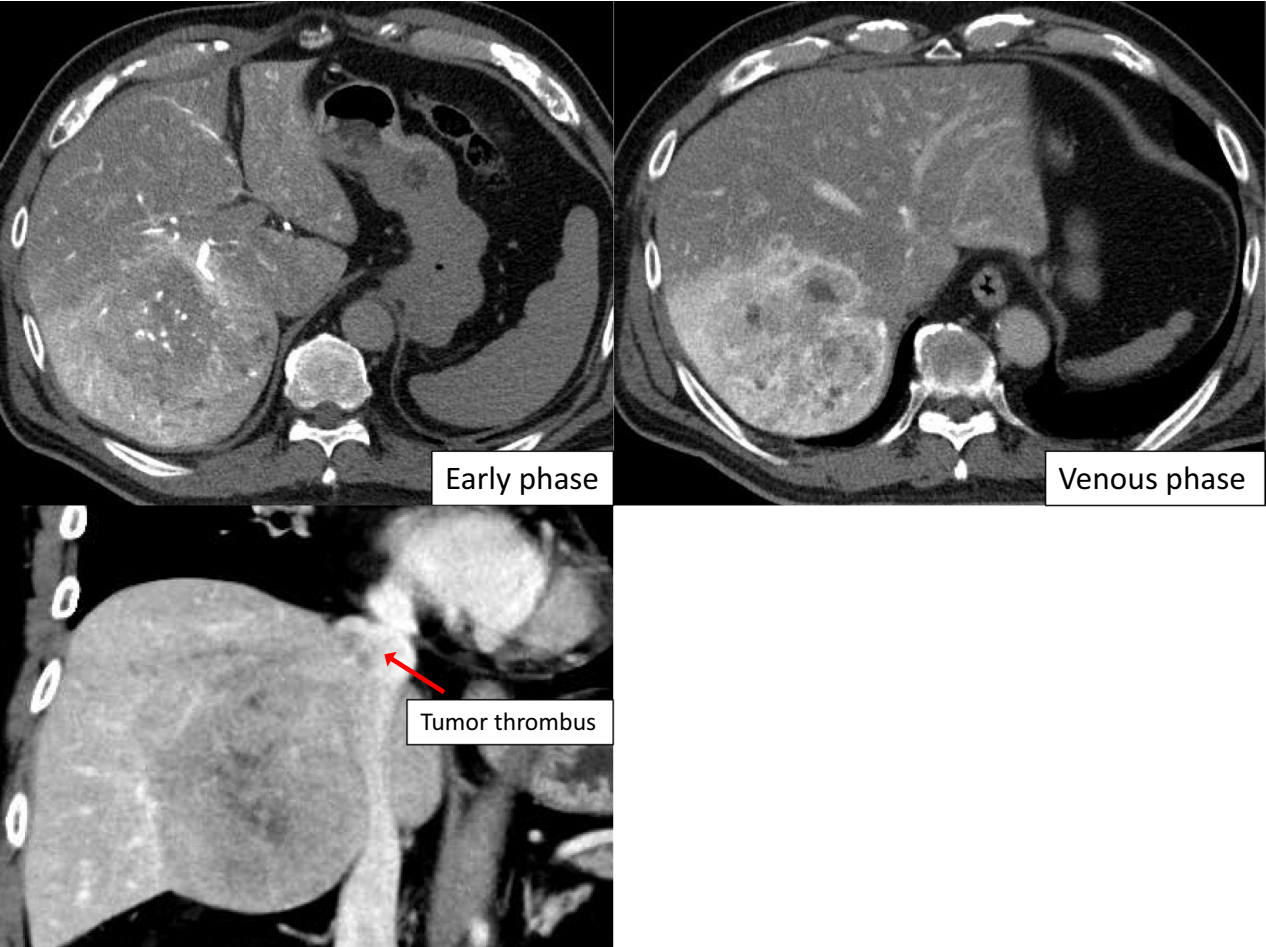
Abdominal computed tomography showed a tumor with tumor thrombus extending to the IVC
